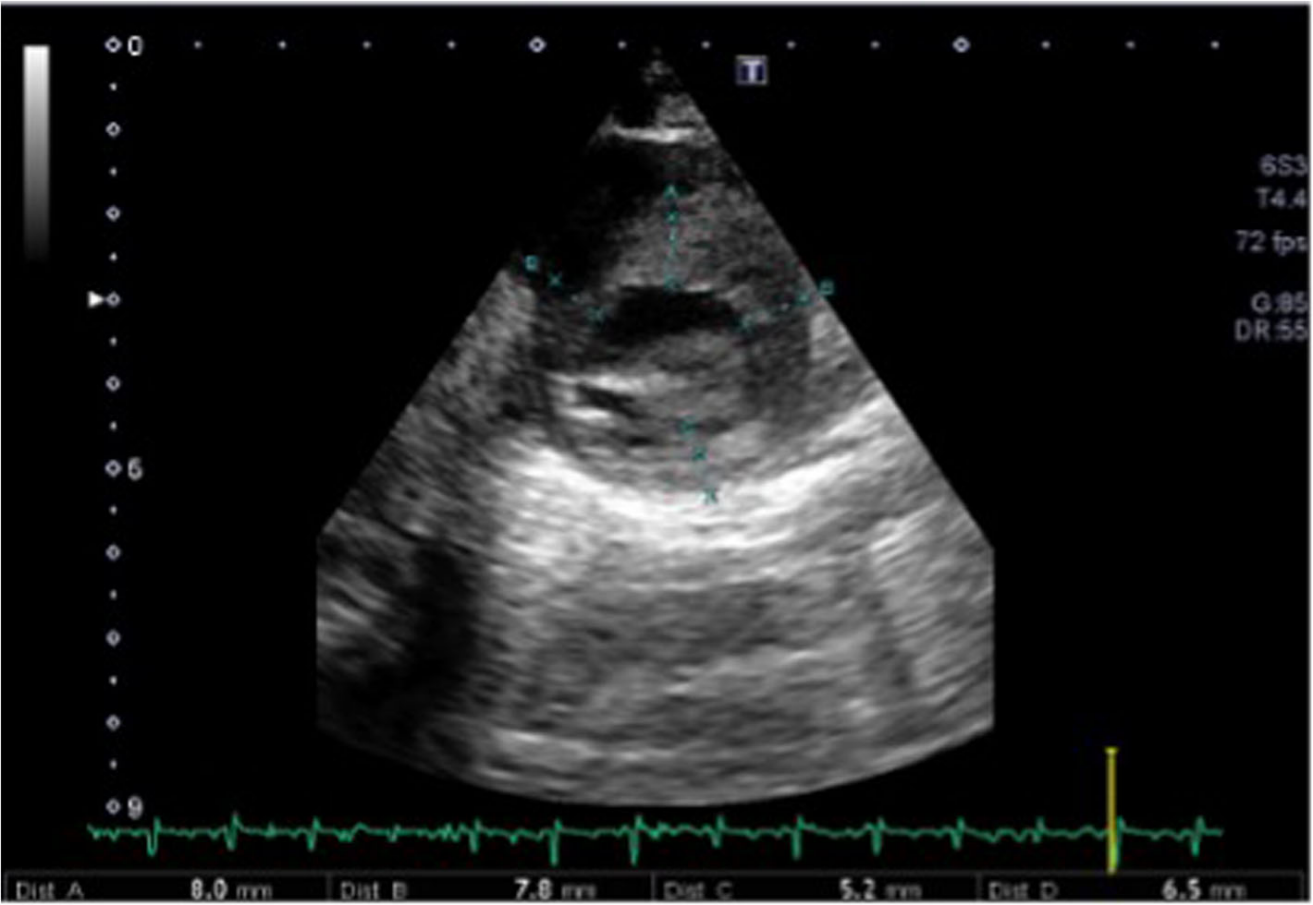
Echocardiogram performed (6 months of age) showed a significant reduction of the interventricular septal hypertrophy (IVSd of 8 mm; z-score 3.01)
